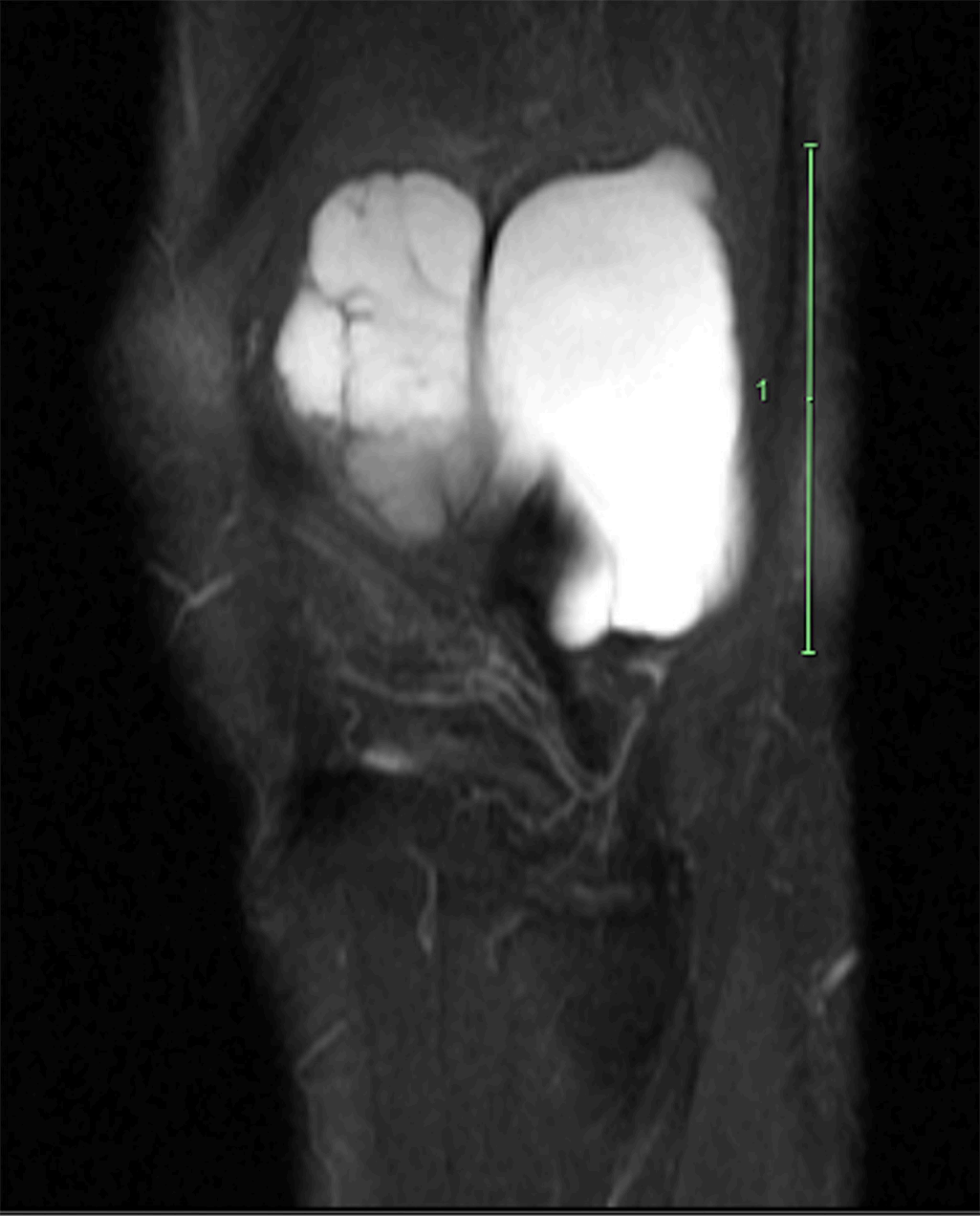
T2 sagittal view MRI without contrast of the left knee depicting multiloculated cystic lesion deep to the IT band.
